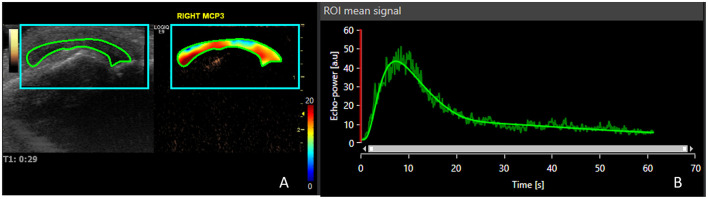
Contrast-enhanced ultrasound quantification using VueBox^®^ software of the same joint in Figure 1. (A) The region of interest is depicted, and the heat map shows the contrast enhancement through the 90 s clip after contrast arrival; (B) time–intensity curve allowing the determination of signal intensity maximum and minimum.
